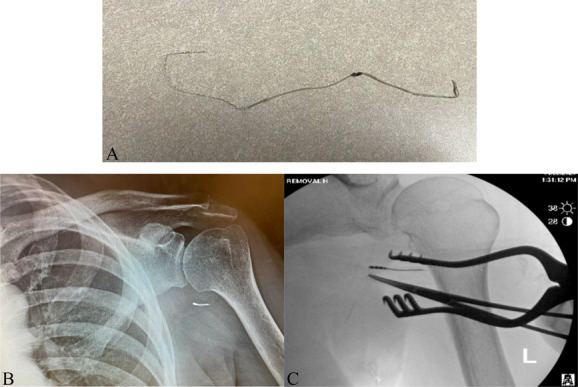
Case 2. (A) Peripheral stimulator lead removed in clinic. (B) X-ray with retained PNS lead fragment. (C) Medial migration of PNS lead fragment during attempted surgical removal in the operating room.
